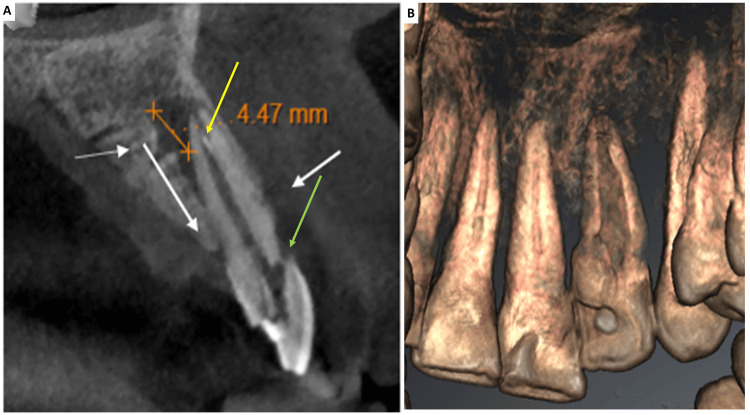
(A) CBCT revealed residual gutta percha fragment (yellow arrow) and a labio-incisal perforation (green arrow) in the coronal aspect and confirmed by (B) 3D reconstruction CBCT: cone-beam computed tomography, 3D: three-dimensional
